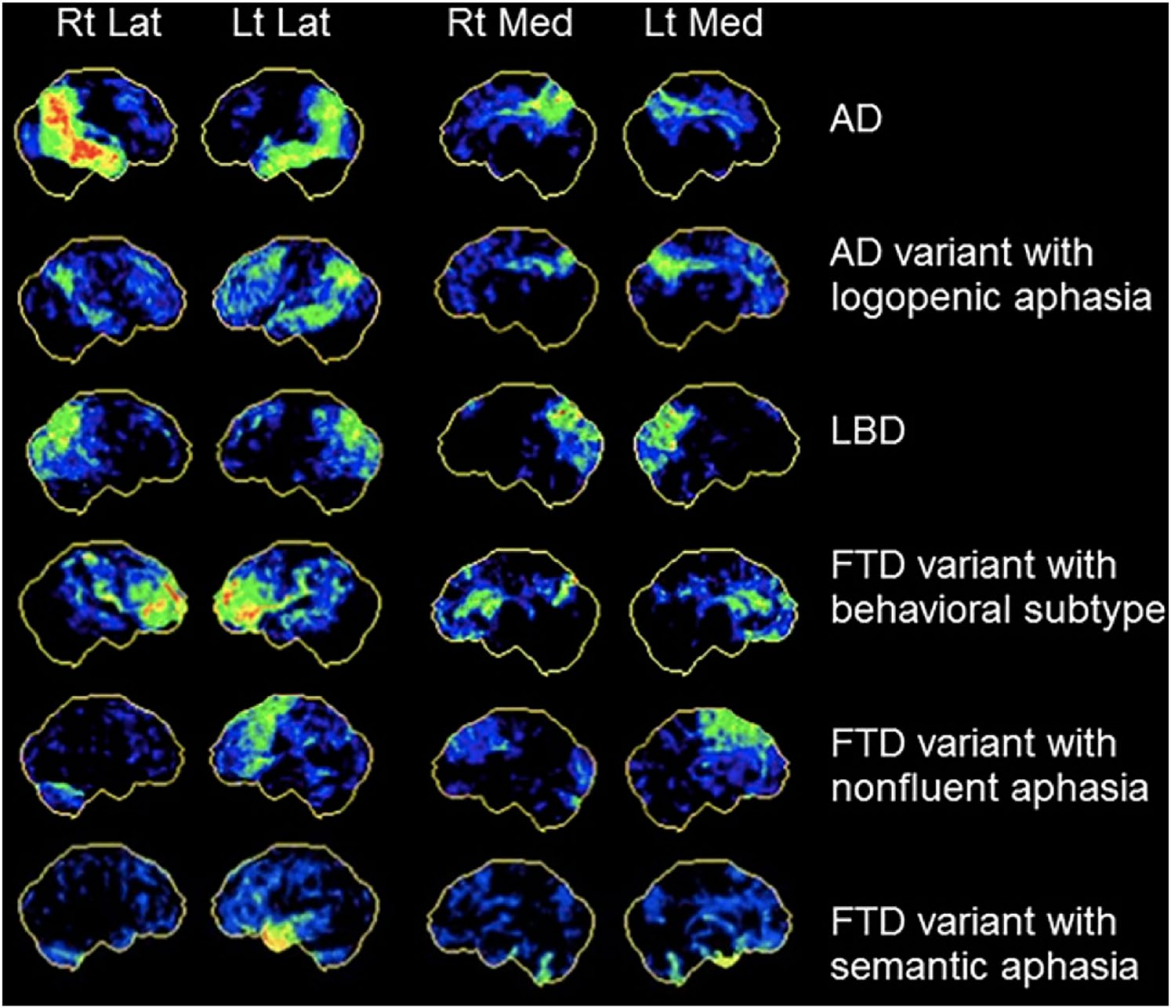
18F-FDG PET scan patterns for focal-onset dementias using Neurostat 3D-SSP where *Lat* lateral, *LBD* Lewy body dementia, *Lt* left, *Med* medial, *Rt* right. Reproduced with permission from Taswell et al. [20]
